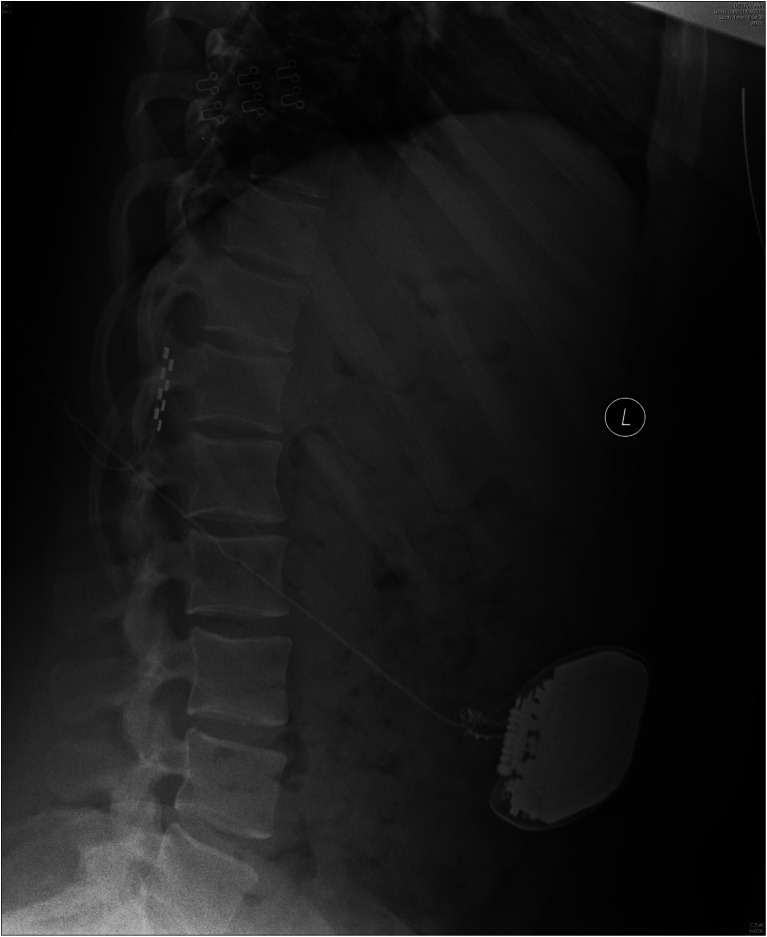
Lateral plain abdominal X-ray showing multiple twists of the connecting wires adjacent to the IPG
